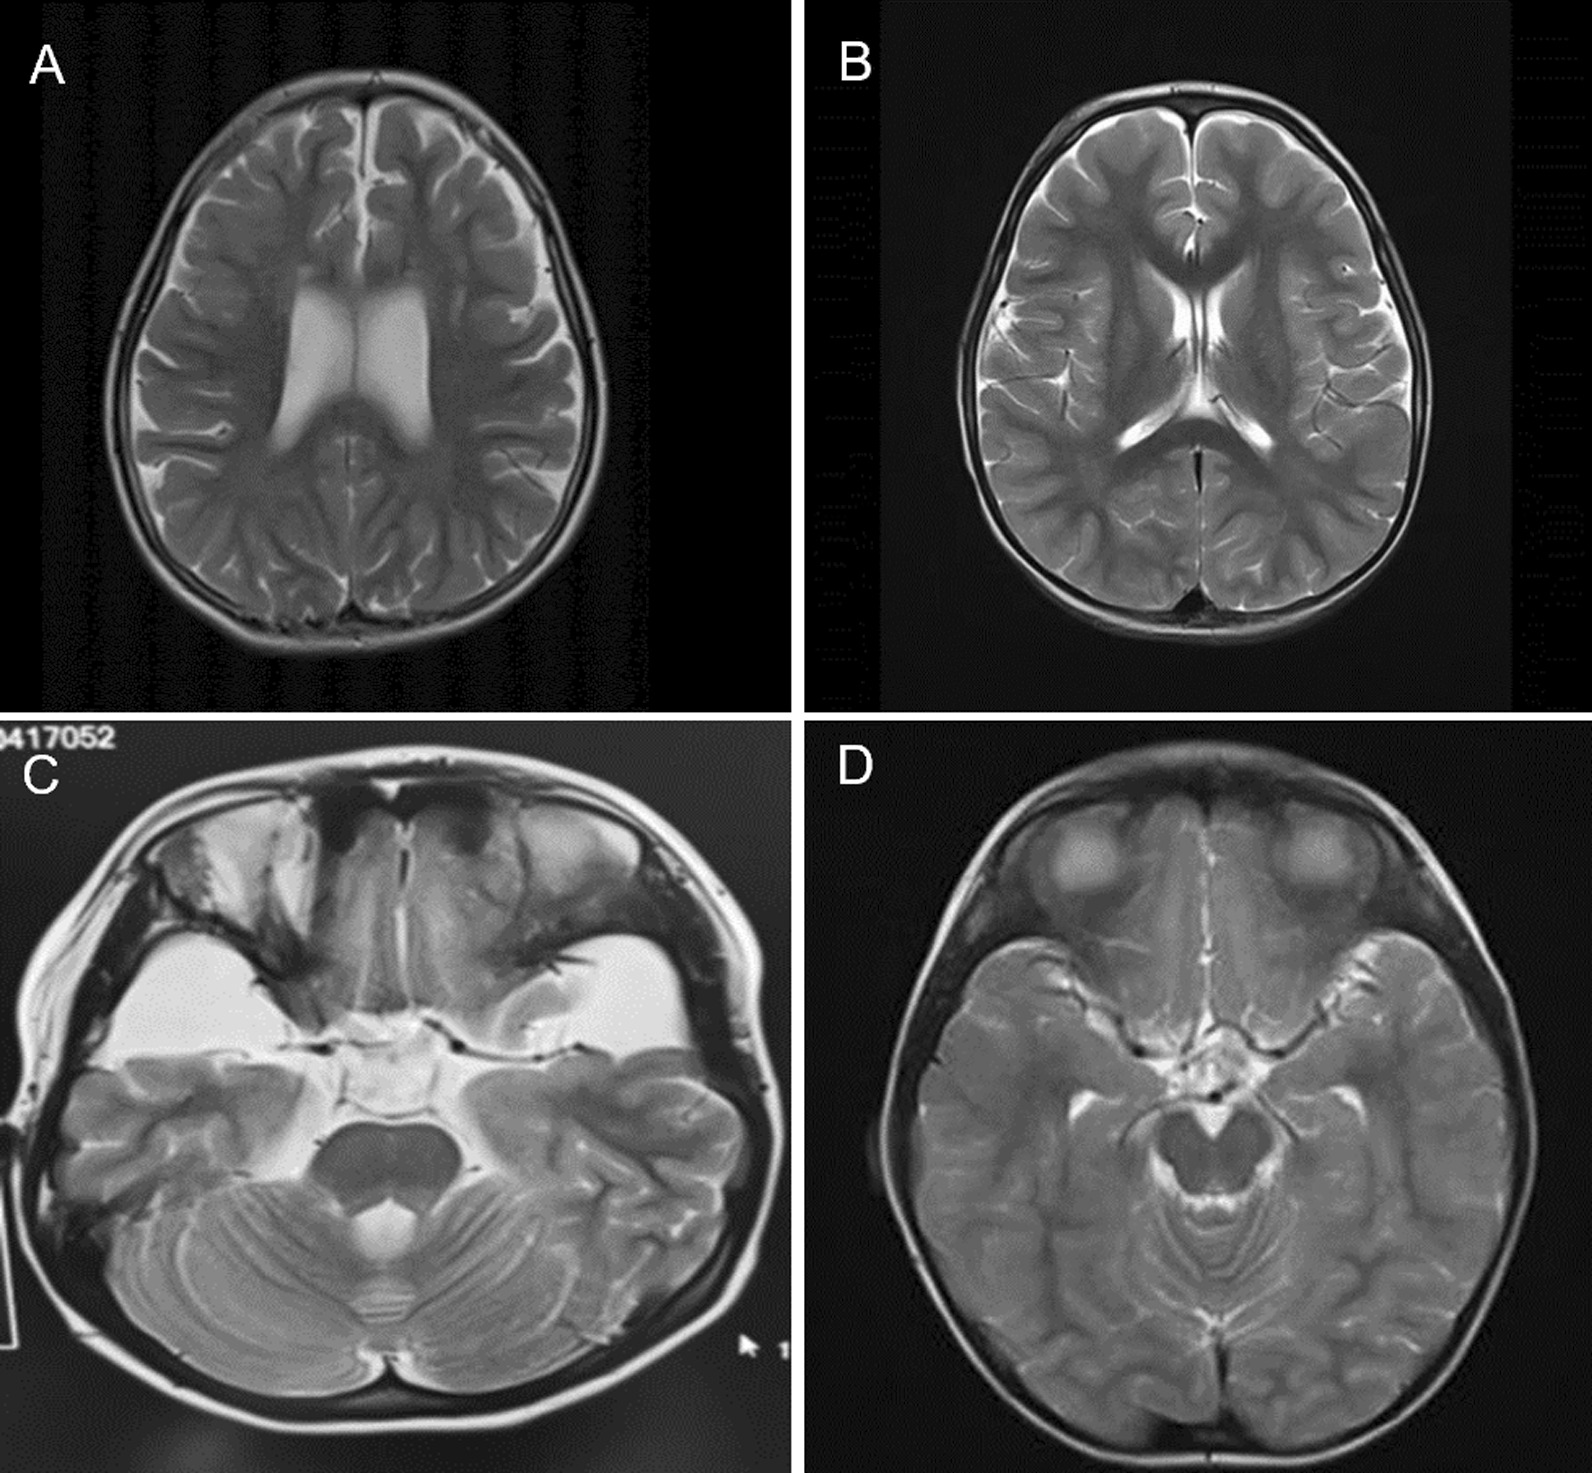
Characteristics of cranial MRI in a patient with methylmalonic acidemia (A, B) and a patient with glutaric acidemia (C, D). A: Iso-T1 and long T2 signals shown in the white matter of the frontal lobes, bilateral frontal white matter demyelination enhancement and mild dilatation of the lateral ventricles (at 3 months of age). B: Normal (at 2 years of age). C: The bilateral temporal subarachnoid space was significantly enlarged (at 3 months of age). D: Normal (at 2.5 years of age)
